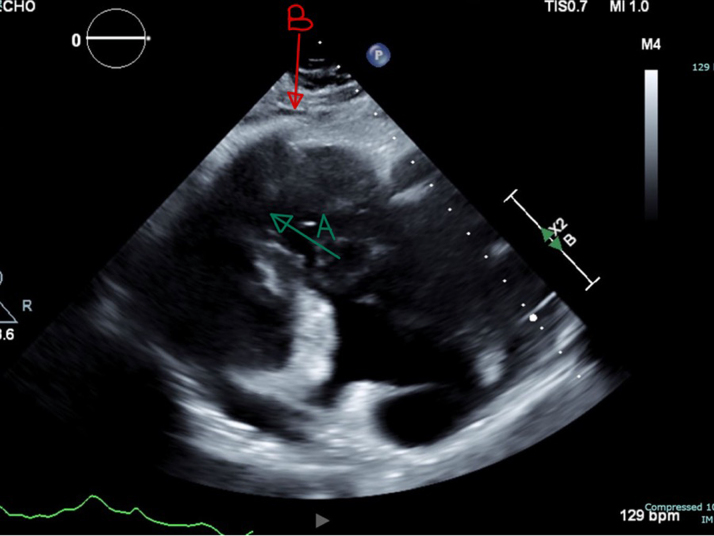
Transthoracic Echocardiography Demonstrating Right Ventricular Mass Right ventricular outflow view on transthoracic echocardiography demonstrating a large, immobile right ventricle (RV) mass (A) and occupying most of the RV cavity and extending into the right ventricular outflow tract (RVOT). The mass is echogenic and attached to the anterior RV wall (B), with no evidence of thrombus or vegetations. Findings are suggestive of primary cardiac lymphoma (PCL).
